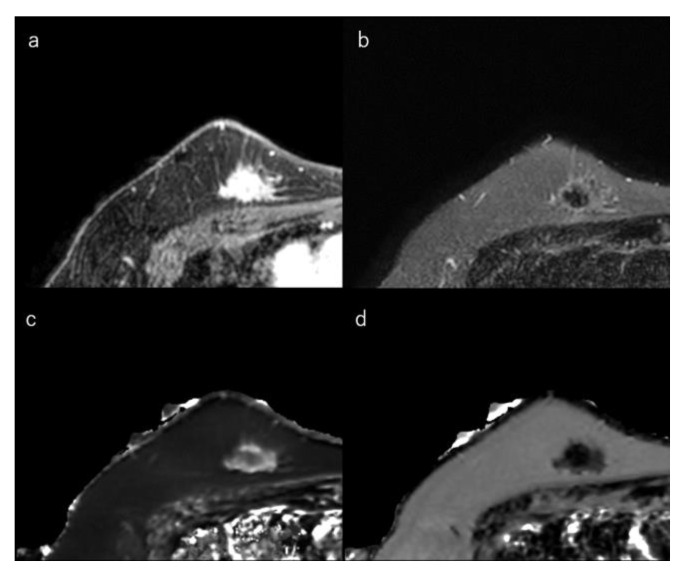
(a) Dynamic contrast-enhanced MR image in a 50-year-old woman with luminal-A type breast cancer shows a spiculated mass. (b) Short-tau inversion recovery image shows a low signal mass. (c) T1 map (window width/centre = 1400/2400 ms) shows an intermediate signal mass; Mean T1 of the mass is 986 ms. (d) T2 map (window width/centre = 160/240 ms) shows a low signal mass; mean T2 of the mass is 62 ms.
